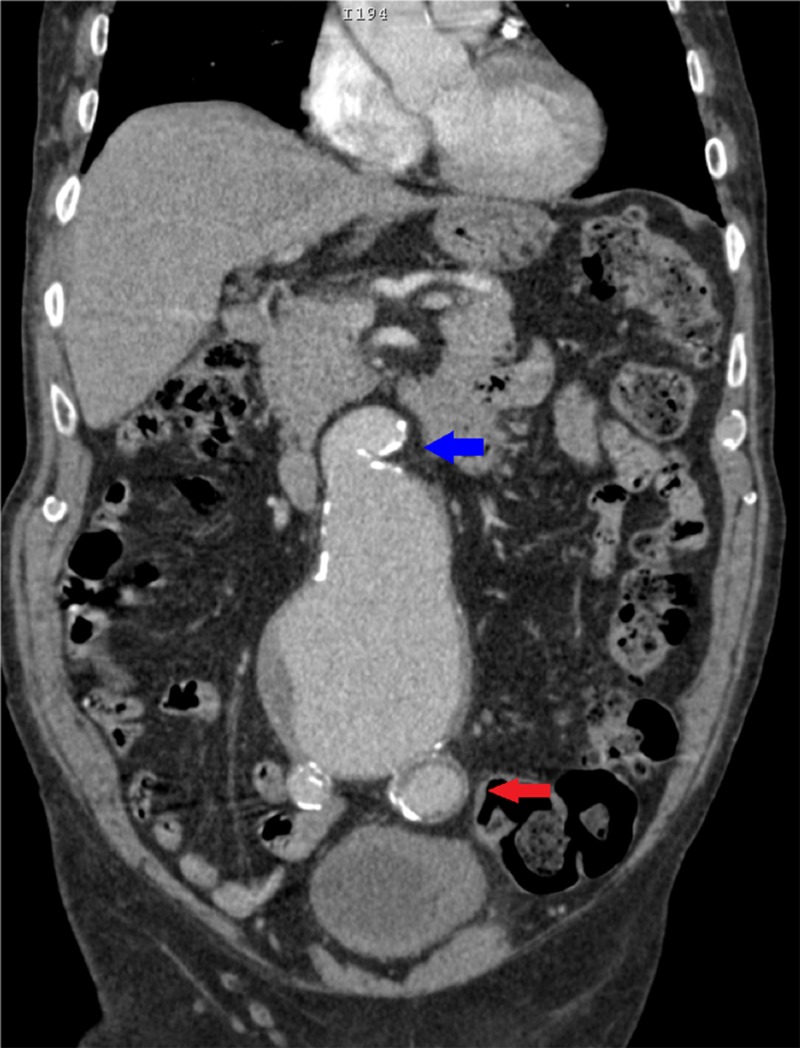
Coronal view of a contrast-enhanced CT of the abdomen and pelvis showing a 9.5 cm infrarenal AAA with a 90-degree proximal neck angulation (blue arrow) and peripheral mural thrombus. Additionally, there is also a 3.3 cm left common iliac artery aneurysm (red arrow). AAA: abdominal aortic aneurysm; CT: computed tomography
